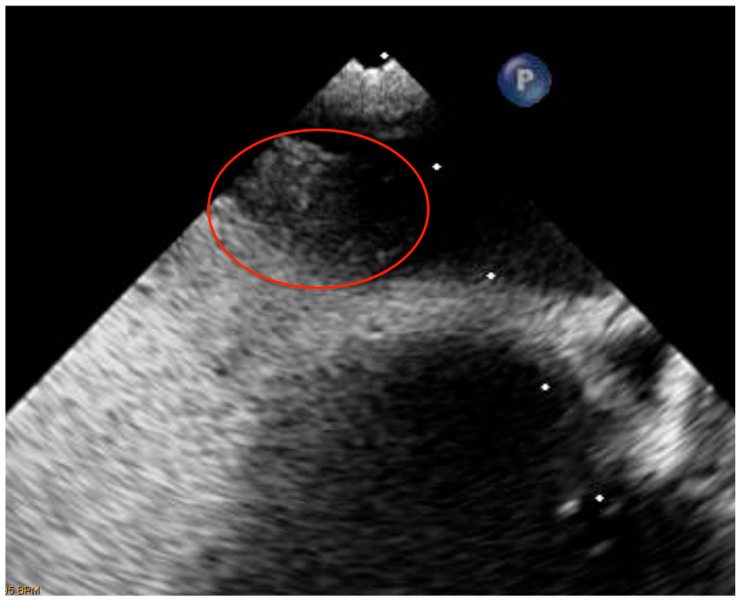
The echocardiographic image displays a secundum atrial septum defect with patent foramen ovale (PFO).
